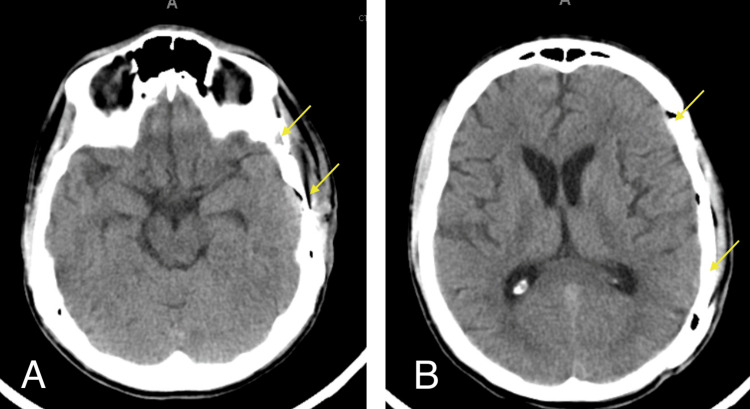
Axial head CT after cranioplasty (yellow arrows)
